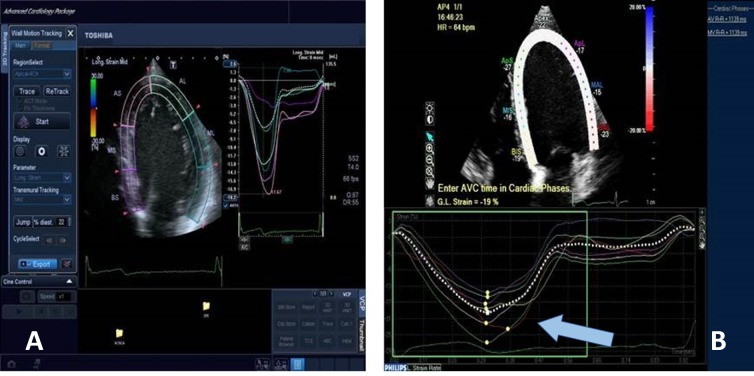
Figure showing the assessment of global longitudinal strain by speckled tracking echocardiography A) The graph represents strain rate (%) over time (in seconds) in a healthy individual. B) The graph represents strain rate (%) over time (in seconds) in a patient with dilated cardiomyopathy. Note: The decrease in global longitudinal strain in the patient with dilated cardiomyopathy (is indicated by the blue arrow)
